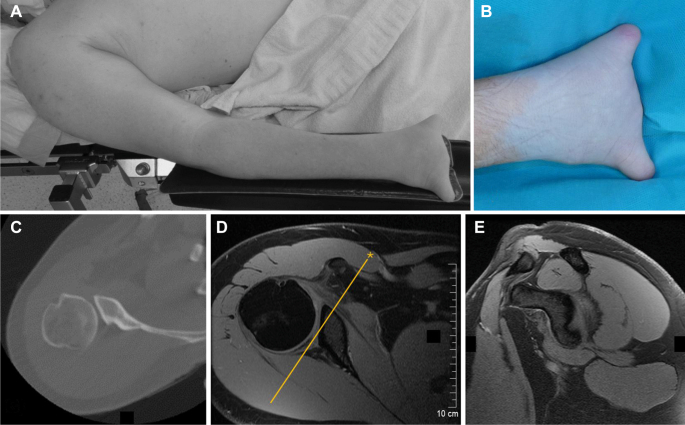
(A and B) Patient exhibited dysmelia with incomplete stump formation but symmetrical shoulder girdle and upper arm musculature. (C) Pre-operative axial CT image showing a posterior glenohumeral instability (Walch type C). (D and E) MRI prior to the first surgical glenoid reconstruction. *CT*, computer tomography; *MRI*, magnetic resonance imaging.
